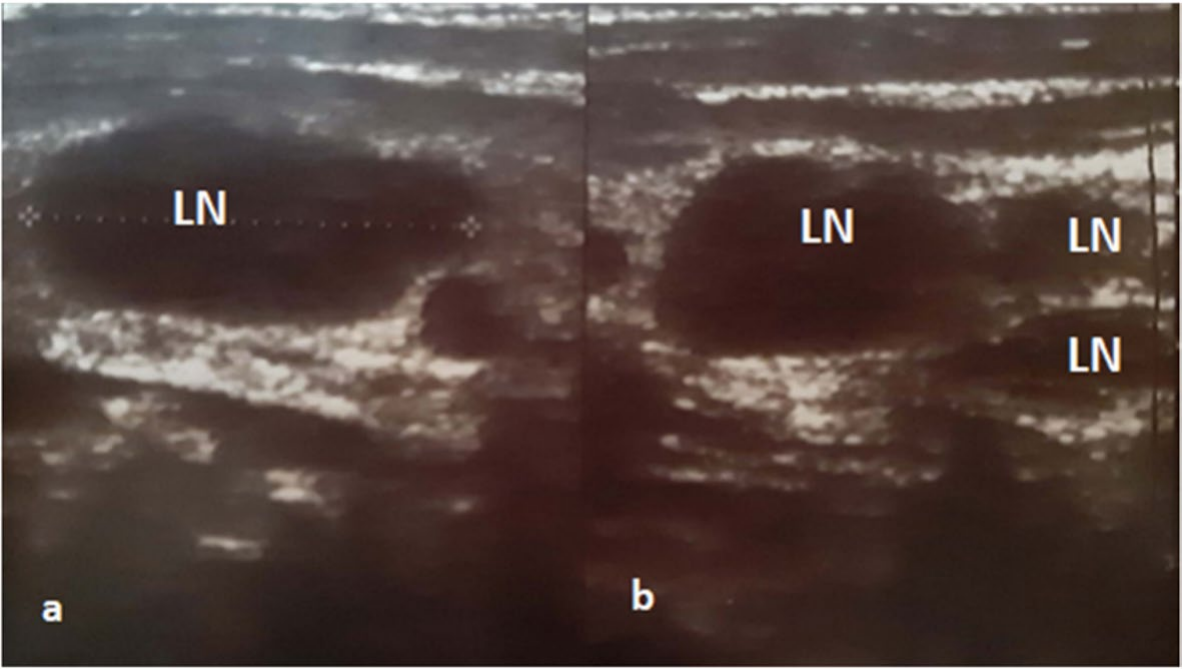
Neck ultrasound prior to delivery. a The largest lymph node. b Multiple lymph nodes (LN) on the right posterior triangle, the largest one measures 10 × 20 mm with loss of fatty core
